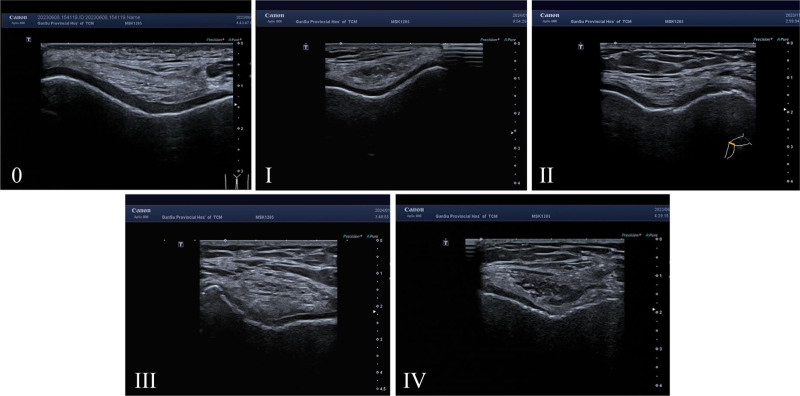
Ultrasound image characteristics of grades 0 to IV cartilage injury.
